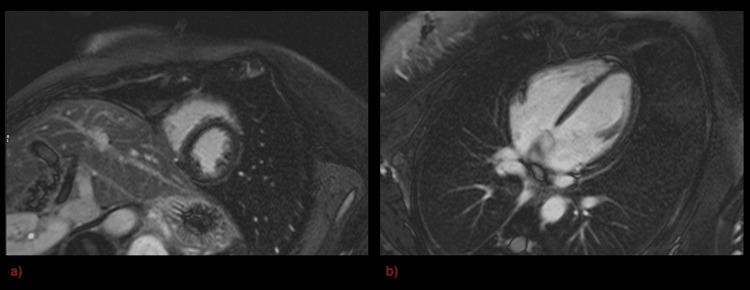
Repeat gadolinium enhanced cardiac MRI
